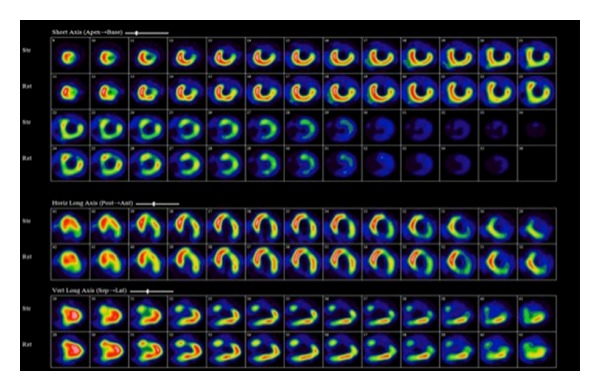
Baseline Tc-99m SPECT myocardial perfusions scan from CRT candidate demonstrating a fixed perfusion defect involving anterior and anterolateral wall consistent with transmural scar. Intraoperative testing demonstrated a high pacing threshold at anterolateral LV lead position; excellent pacing threshold was obtained from a posterolateral coronary sinus branch.
